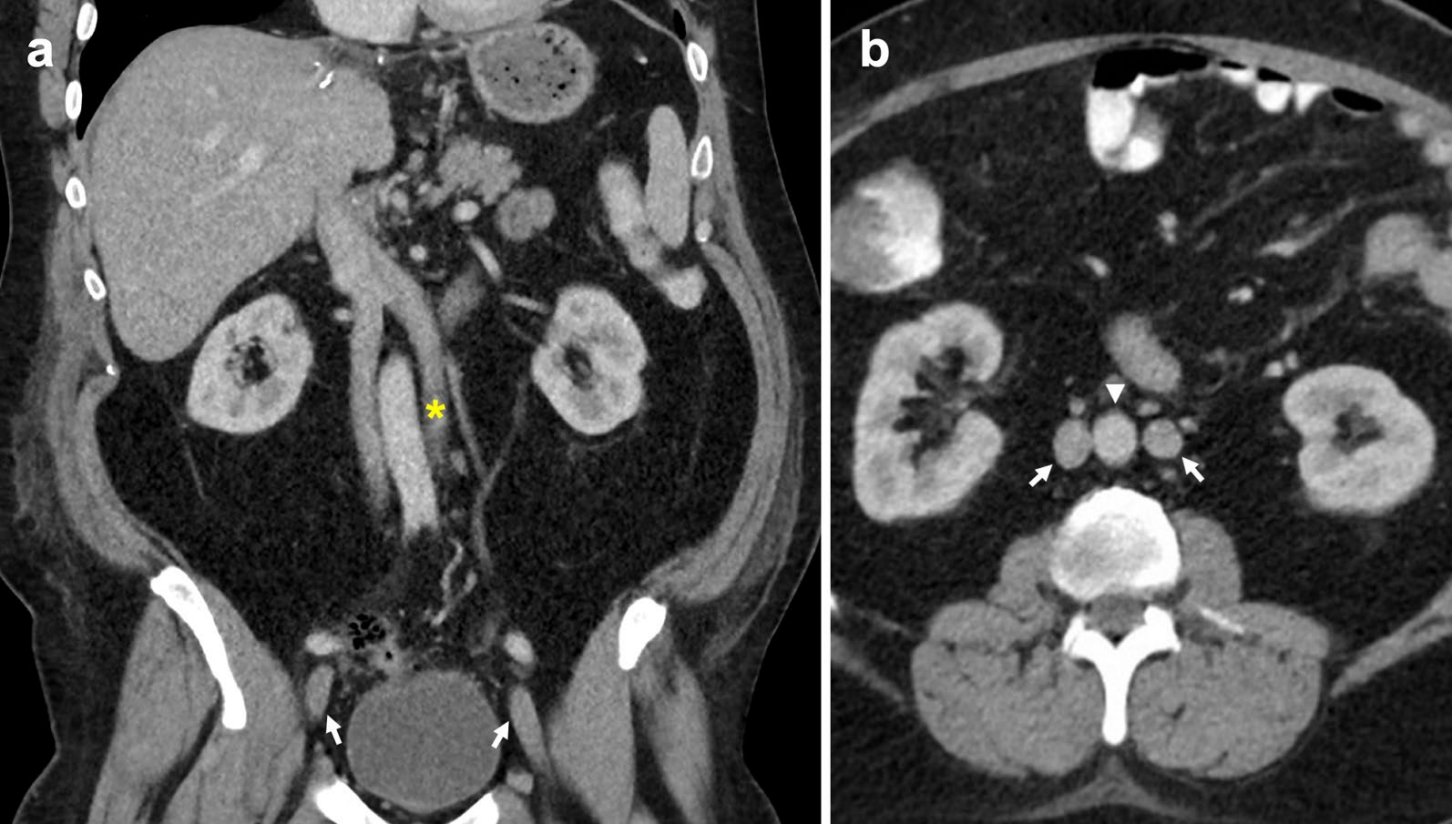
Duplicated IVC with symmetrical caliber. a Contrast-enhanced coronal CT shows a duplicated IVC (asterisk) with symmetrical caliber draining into the suprarenal IVC. Bilateral external iliac veins (arrows) both drain into cavae on their respective sides. b Axial CT image shows the paired IVC trunks (arrows) positioned on either side of the abdominal aorta (arrowhead)
